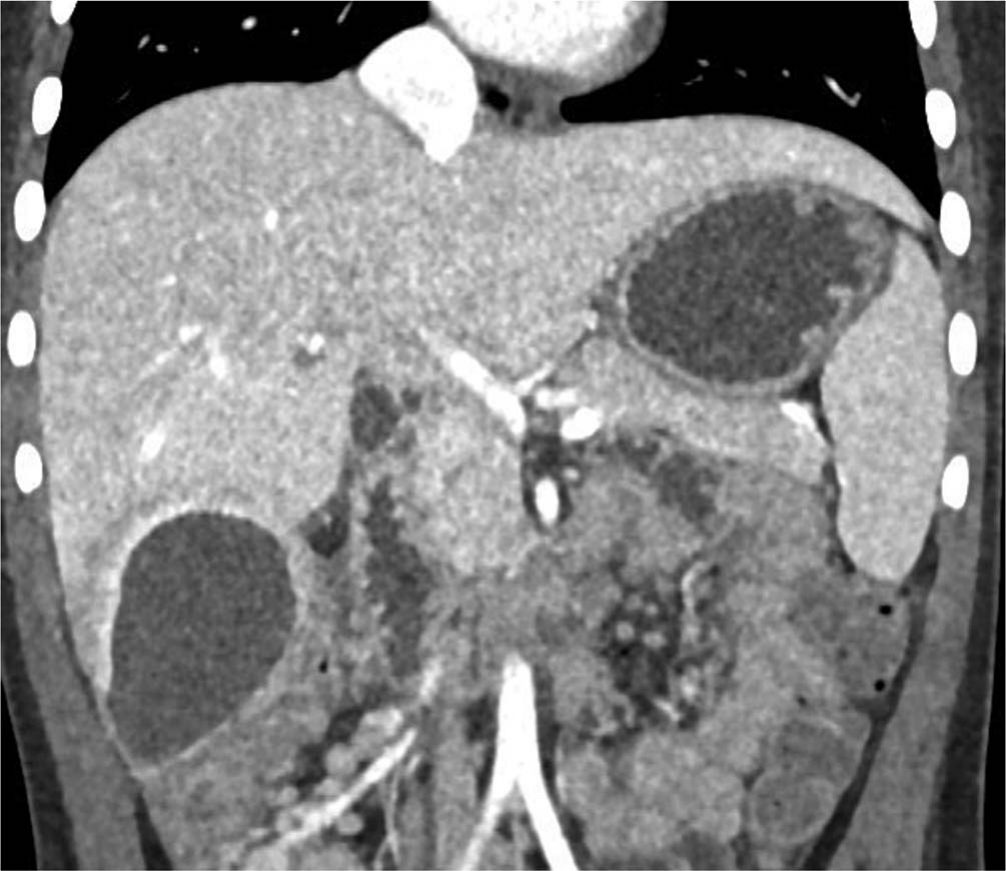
CT coronal view of a subhepatic abscess.
